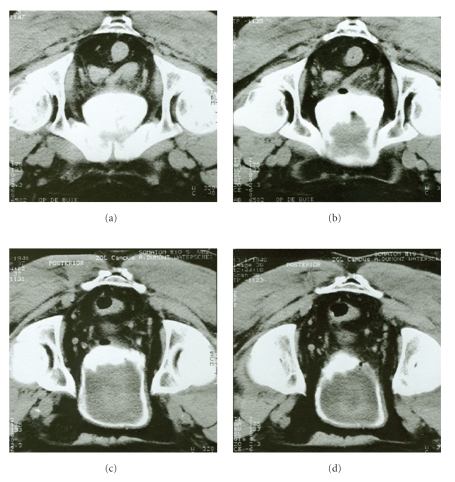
Contrast enhanced CT-scan of the abdomen (prone position), showing a large bladder mass, protruding the bladder and almost completely filling up the bladder lumen.
